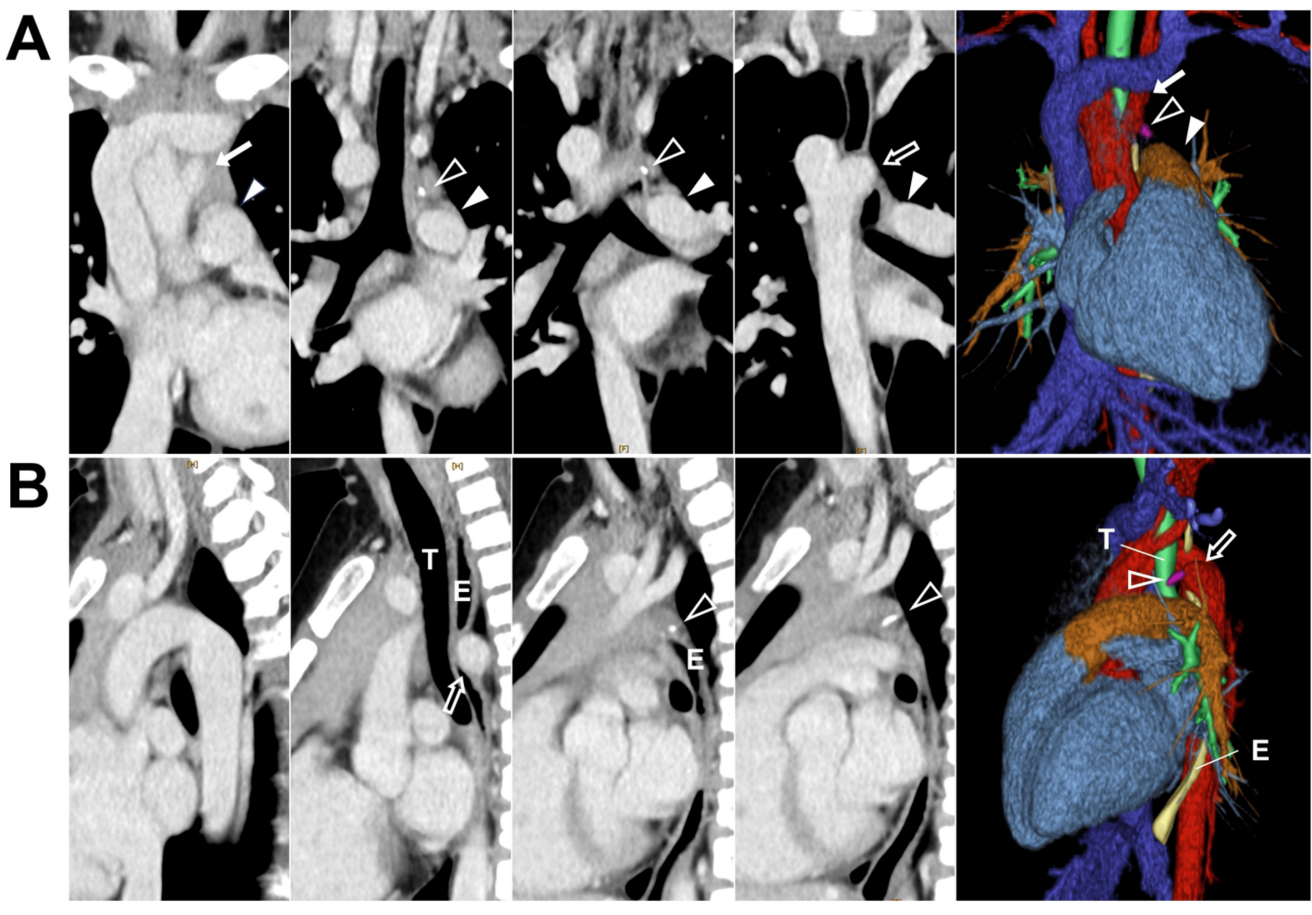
Contrast-enhanced cervicothoracic CT findings of ligamentum arteriosum calcification A. Coronal imaging. A linear, high-density lesion was observed between the Kommerell diverticulum and the pulmonary artery. Arrows indicate the left subclavian artery, arrowheads indicate the pulmonary artery, open arrow indicates the Kommerell diverticulum, and open arrowheads indicate calcification of the ligamentum arteriosum. B. Sagittal imaging. The Kommerell diverticulum can be seen compressing the trachea and esophagus from behind. Open arrows indicate Kommerell diverticulum, and open arrowheads indicate calcification of the ligamentum arteriosum. T, trachea; E, esophagus
